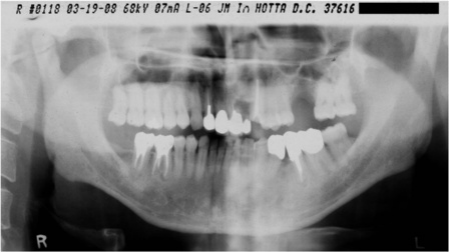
Case 2. March 2008. Panoramic X-ray film taken before #26 GBR
